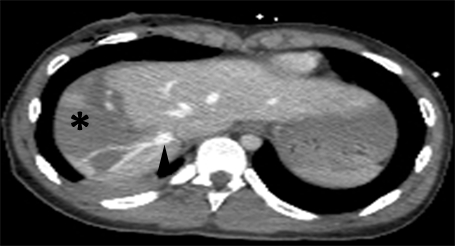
Abdominal CT. Degree Ⅳ liver laceration. Black arrow – right hepatic vein.
